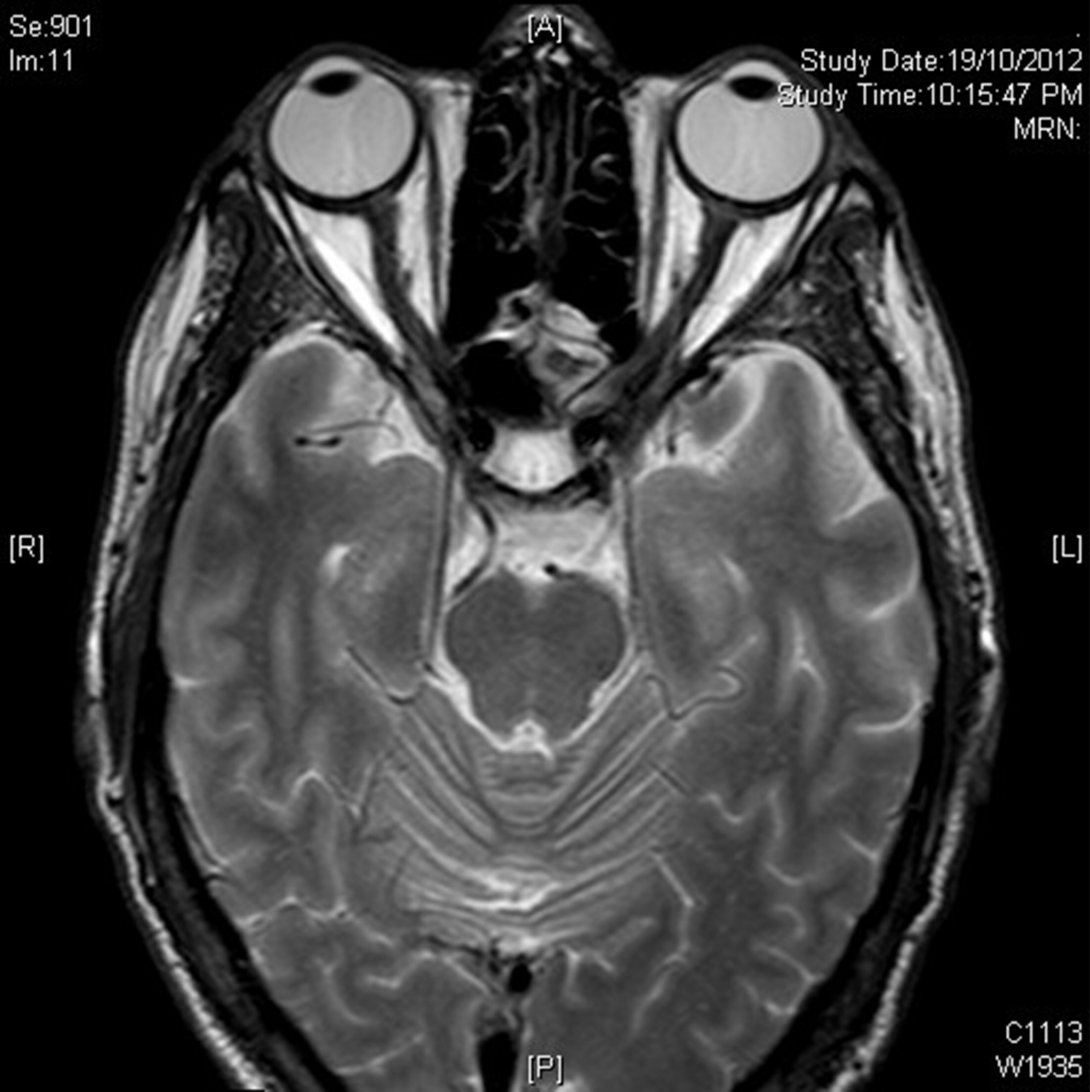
MRI scan of the orbit showing no evidence of active neuritis or infiltration of the optic nerves.
